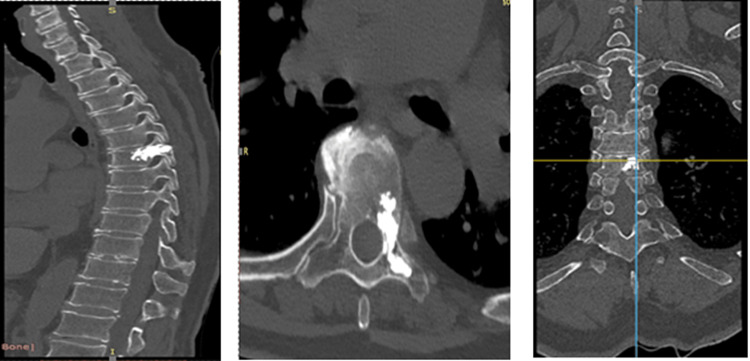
Computed tomography images control: (A) sagittal, (B) axial, and (C) coronal. The images show the placement of the surgical center after the pedicle without apparent effusions.
